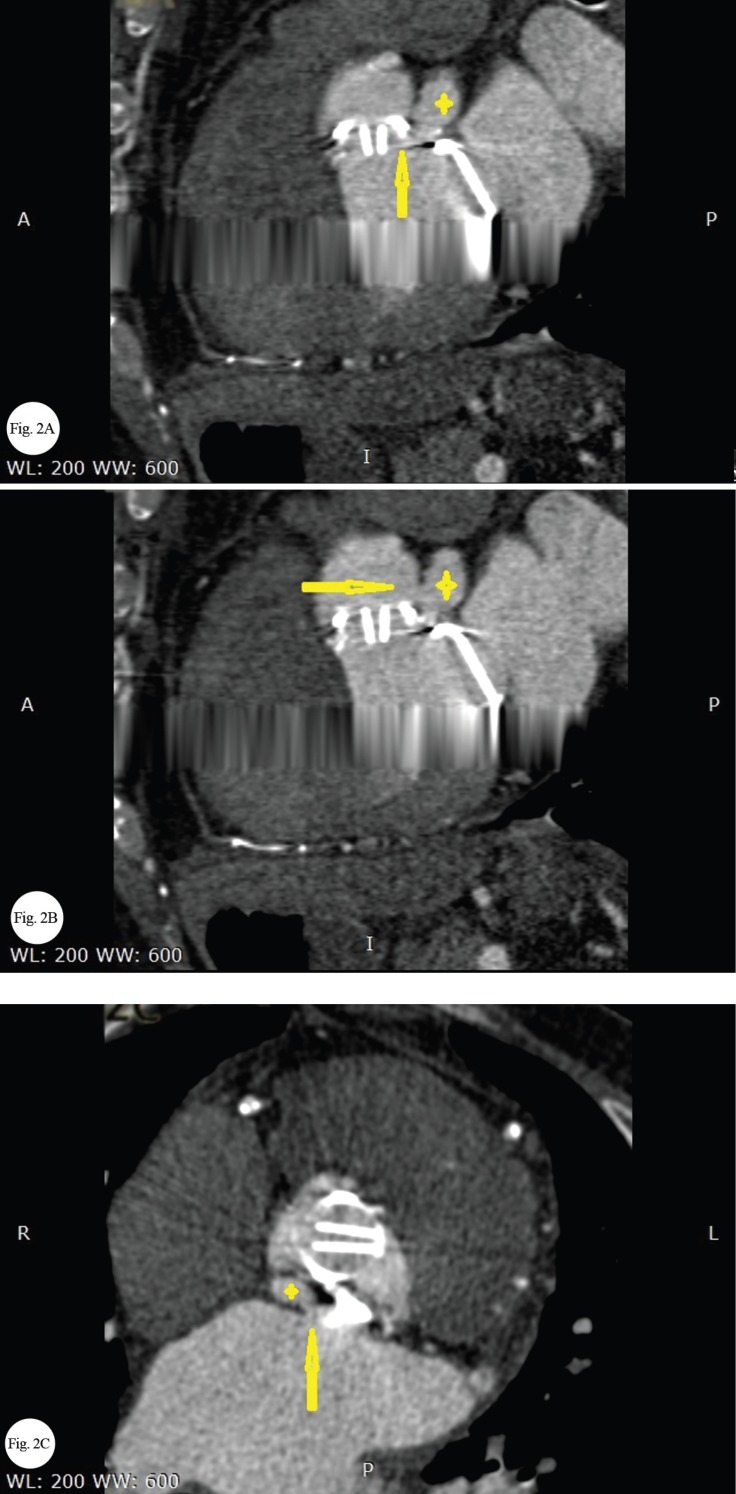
Cardiac computed tomography angiography, showing the pseudoaneurysm (4-point star) with (A) a connection to the left ventricular outflow tract (arrow), (B) the ascending aorta (arrow), and (C) the left atrium (arrow)
